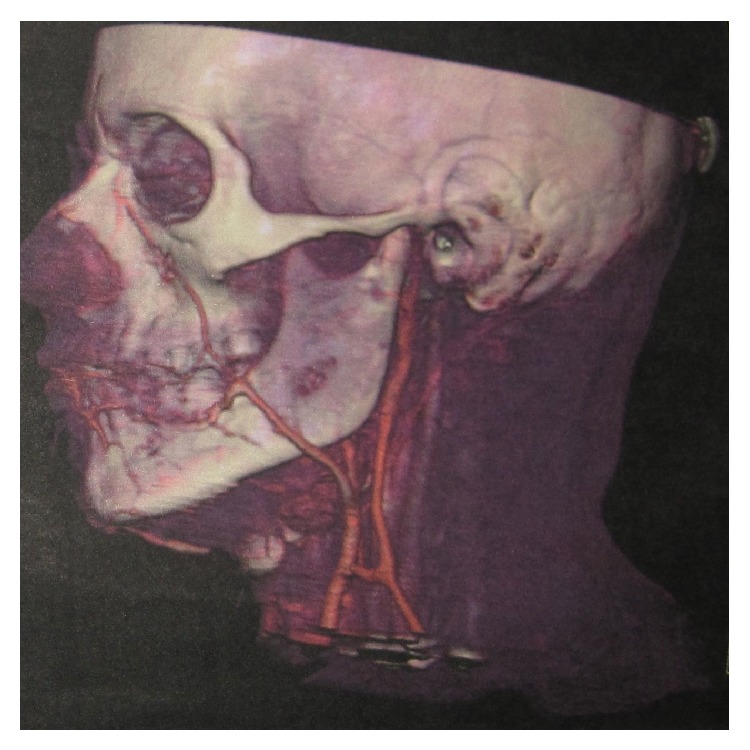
8-year follow-up angio-CT.
